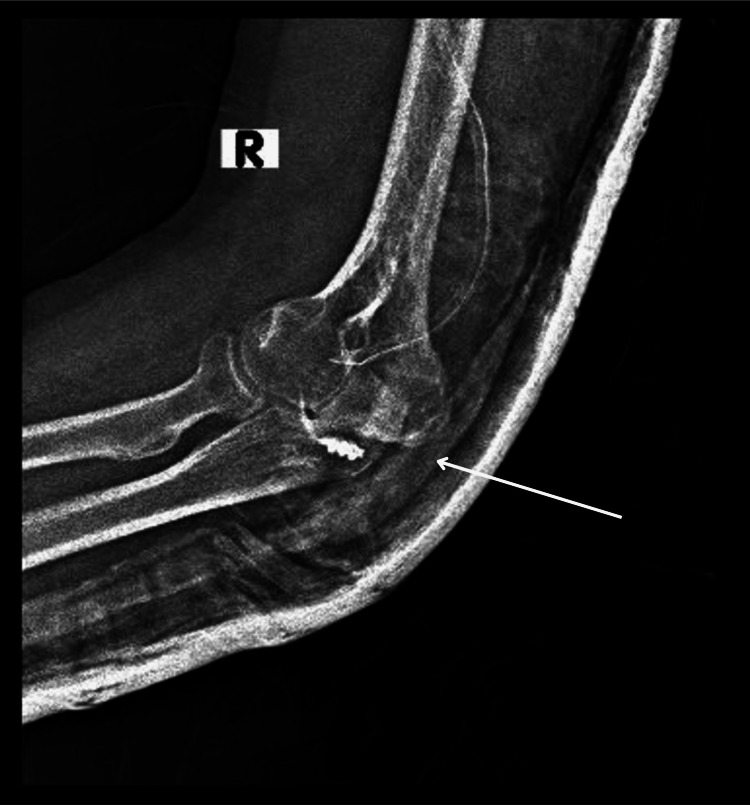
The post-operative X-ray antero-posterior (AP) view showing suture anchor fixation of triceps with heterotopic bone removed
